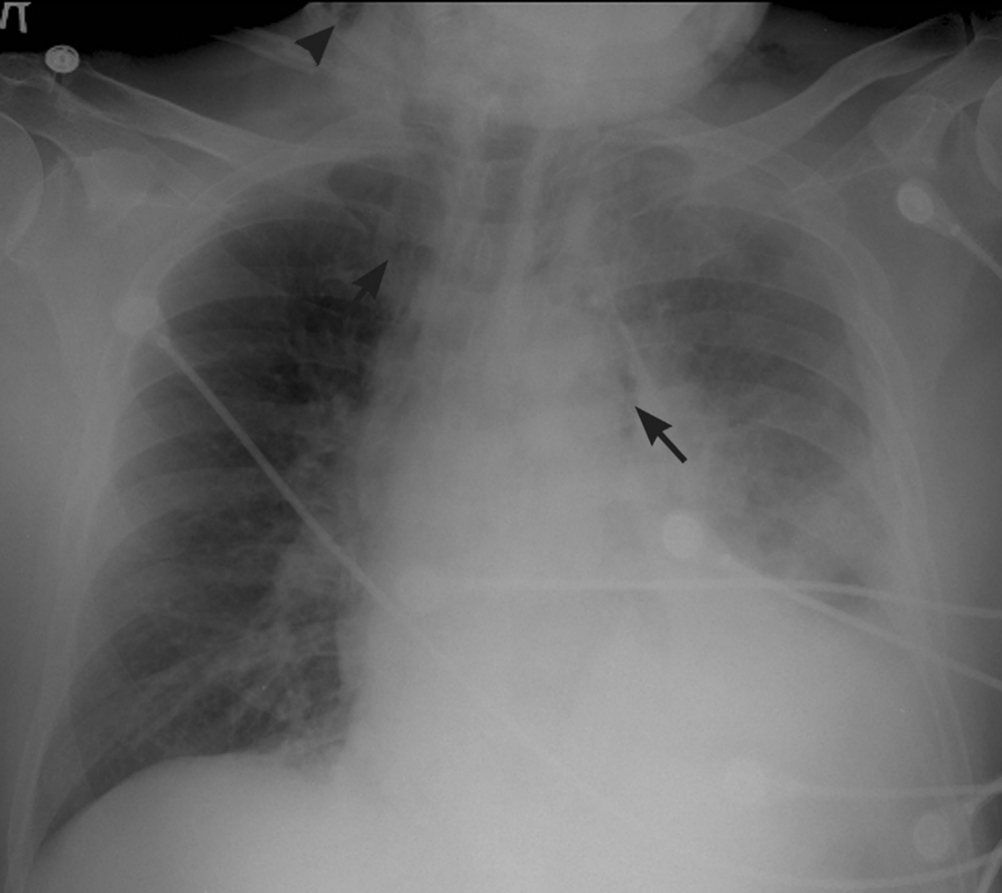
Portable anteroposterior chest radiograph shows pneumomediastinum (arrows) and soft tissue emphysema at the base of the neck (arrowhead). A denture lodged in the oesophagus is obscured by the overlying soft tissues and technique.
